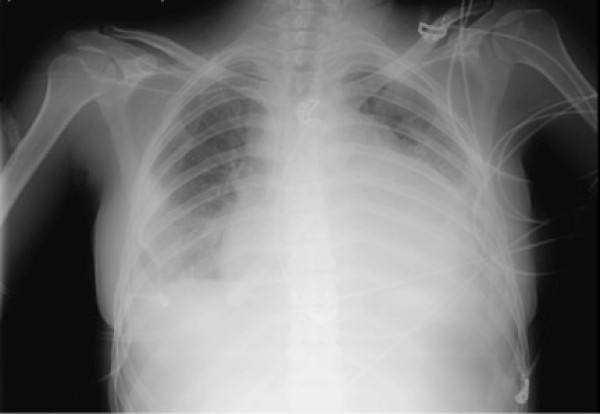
Chest roentgenogram showing cardiomegaly and right pleural effusion.
